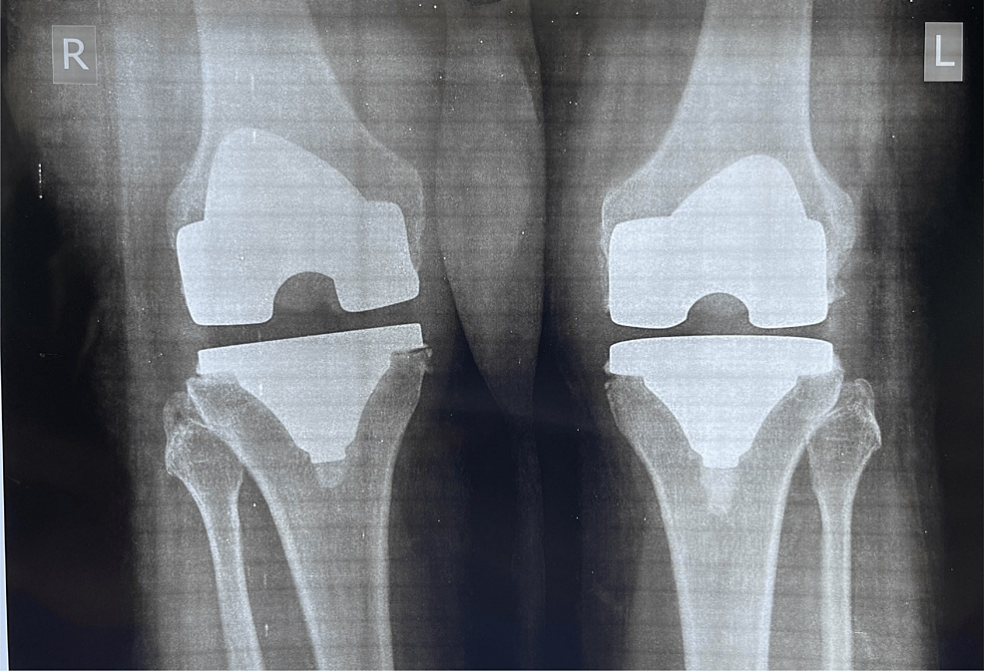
Three months postoperative weight-bearing radiograph showing well-balanced knees
